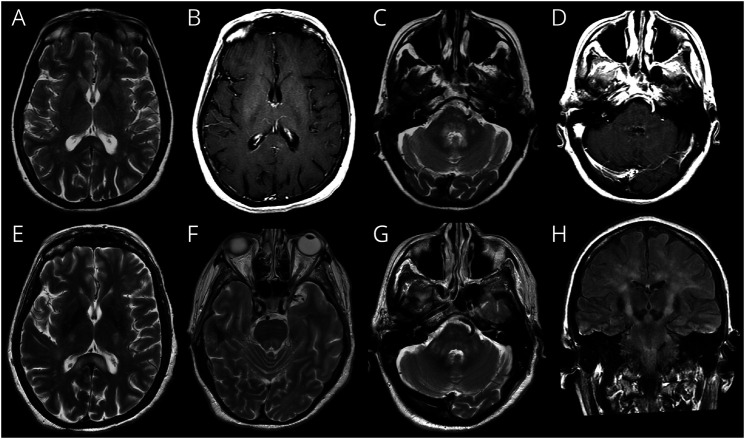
MR Imaging in a Patient With HAM and HTLV-1–Related Encephalopathy MRI of a female patient with HAM presenting with rapidly worsening weakness, headaches, a 6th nerve palsy, and a high CSF PVL of 120%. Axial T2w (A and C) and postcontrast T1w (B and D) images demonstrates a left splenial lesion that demonstrates faint enhancement (A and B) and further abnormal signal with subtle enhancement around the 4th ventricle in the dorsal pons, middle cerebellar peduncles, and dentate nuclei. After IV corticosteroids, the patients' symptoms improved and the lesions regressed (not shown). Eight months later, after debridement of a sacral decubitus ulcer, the patient had a new episode of deteriorating consciousness with recurrence of the 6th nerve palsy. Axial T2w (E,F,G) and coronal FLAIR (H) images showed recurrent and more extensive splenial (E), dorsal pons, and middle cerebellar peduncle (G) lesions and new extensive confluent abnormal signal along both corticospinal tracts (H) from the precentral white matter, into the internal capsules (E) and pons (G).
